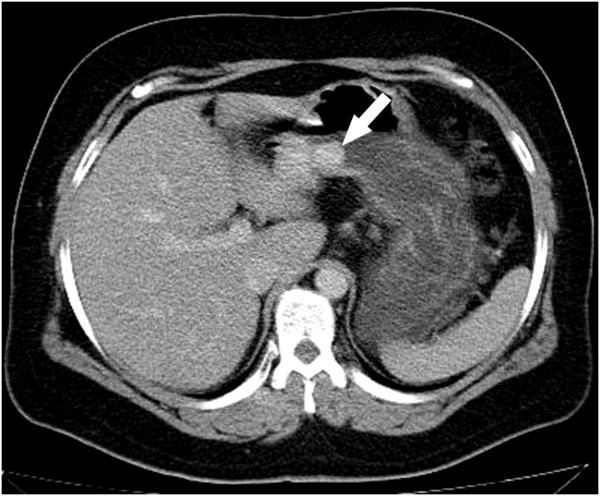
Abdominal computed tomography (CT) scan of the stomach showing the inflammatory myofibroblastic tumor (IMT). Hyperattenuating, focal, polypoid posterior wall thickening was identified near the angular incisure of the stomach.
